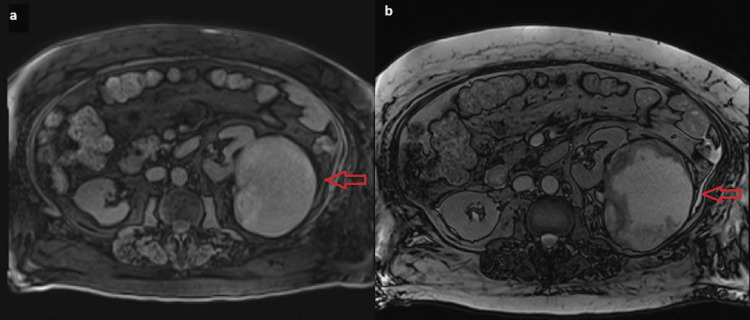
T1 (a) and T2 (b) MRI of the left kidney mass indicated by arrows MRI: magnetic resonance imaging
